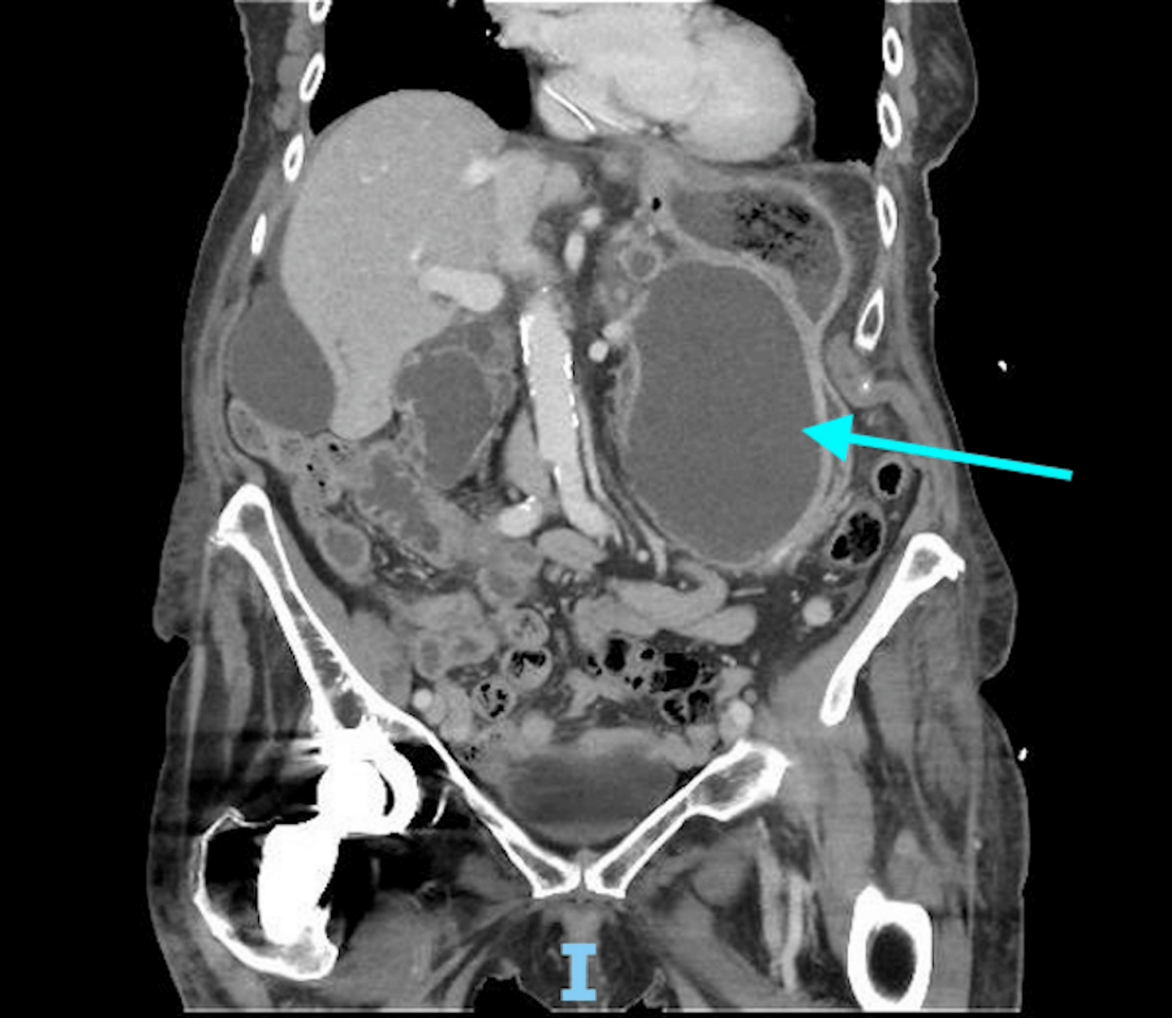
CT of the abdomen and pelvis with intravenous contrast performed on readmission to the tertiary care center. CT: computer tomography.
